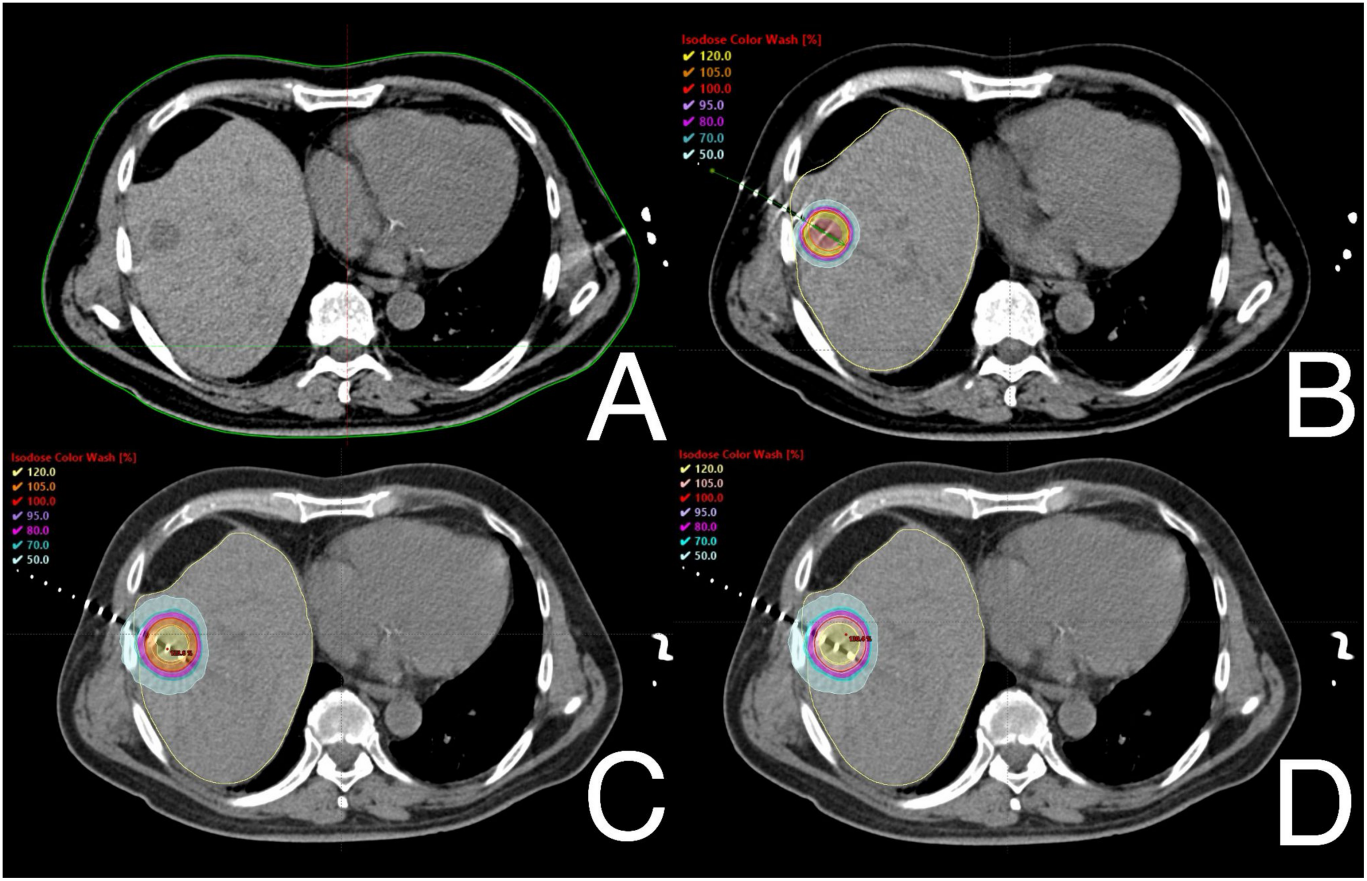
Example of dose distribution profiles within PTVs of one of selected patients with metastasis in fifth liver segment: diagnostic scan taken before treatment (A), CT-BRT (B), SBRTtb (C) and SBRTh (D).
